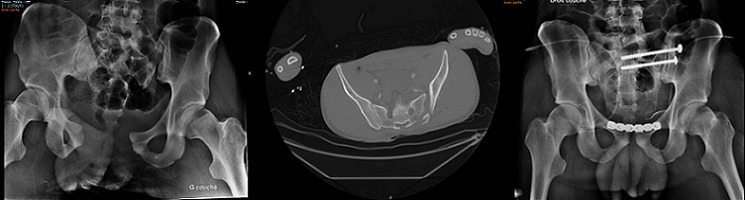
(A) radiographie du bassin montrant la disjonction de la symphyse pubienne et l'ascenssion de l'hémibassin gauche; (B) scanner montrant la disjonction sacro-iliaque gauche; (C) radiographie de bassin de contrôle
